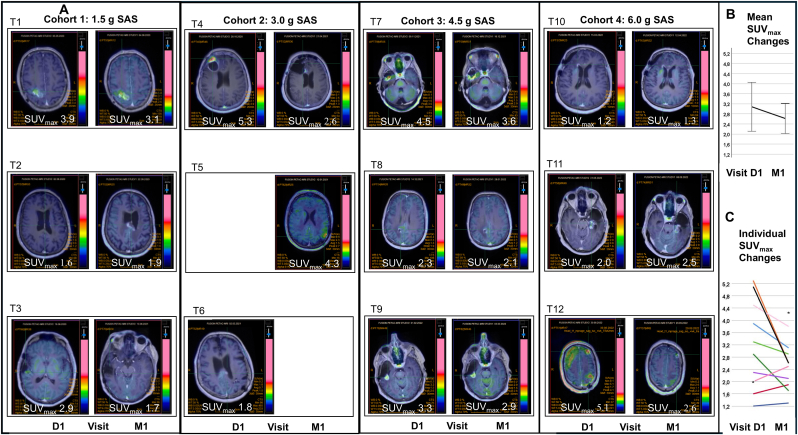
^11^C-Methionine PET images at baseline (D1) and follow-up (M1) for trial participants T1 to T12 (A). Mean changes in SUV_max_ from D1 to M1 (B) and changes in SUV_max_ for all individual trial participants (C). SAS, Sulfasalazine; PET, Positron emission tomography; SUV, standardized uptake volume; D, Day, M, month.
